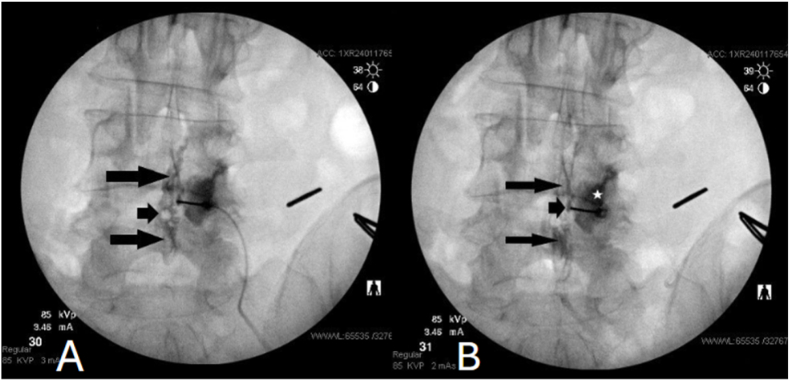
A: Contrast flow pattern in the epidural space following the identification of a “true” loss of resistance during the same injection. The L4-5 facet arthrogram and a small collection of contrast medium in the space of Okada have not changed from previous observations. However, the additional injection of dye reveals a typical epidural flow pattern (long black arrows). The contrast medium exhibits irregular pattern interspersed with patchy vacuolization (short black arrow). B: Contrast flow pattern subsequent to the injection of a therapeutic solution. Anterior-posterior radiograph fluoroscopic image demonstrates a “washing out” of the contrast material in the epidural space (long black arrows). The shape and density of the dye collection in the facet joint (white star) and the space of Okada (short black arrow) remain unchanged.
